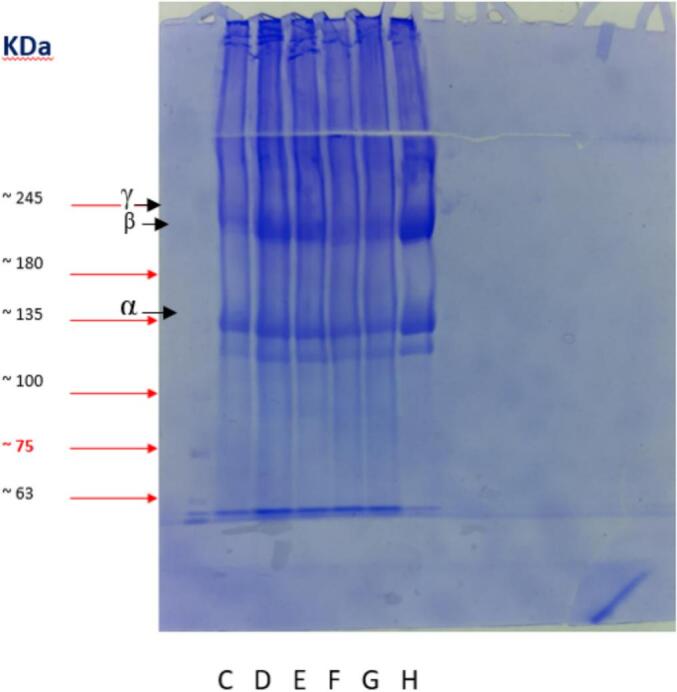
Protein pattern of collagen extracted from beluga skin (C: Choline Chloride- Acetic acid, D: Choline Chloride- Acetic acid 5 min ultrasound, E: Choline Chloride- Acetic acid- 10 min ultrasound, F: Choline Chloride- Acetic acid- 15 min ultrasound, Choline Chloride- Acetic acid- 20 min ultrasound).
